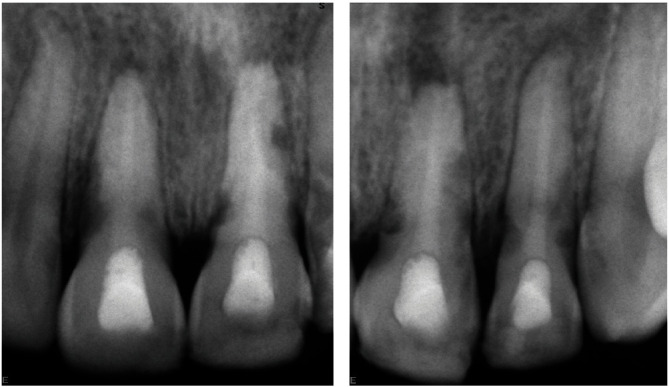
Post-treatment radiographs. (a) Root canal filling in teeth number 11 and 21. (b) Root canal filling in teeth number 21 and 22.
